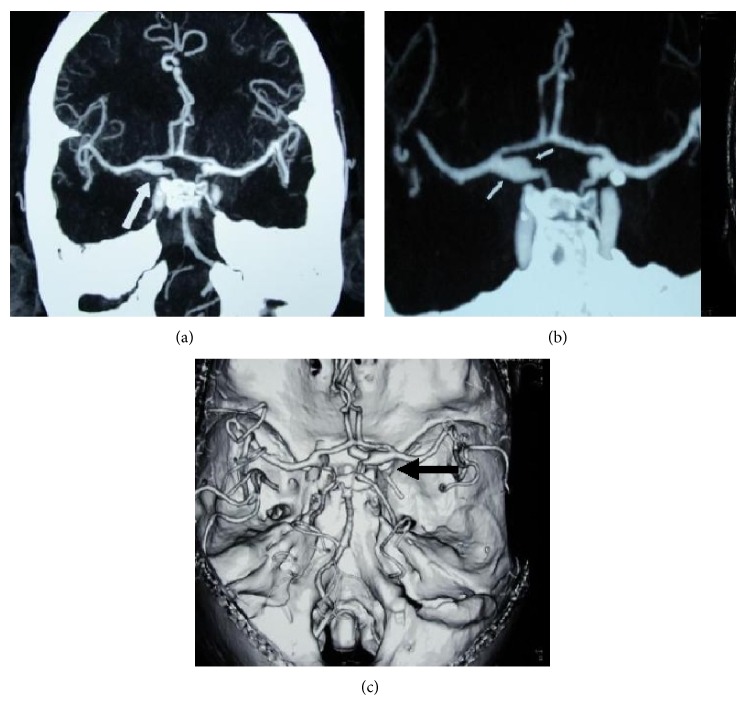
CTA brain showing PCom artery aneurysm. (a) CTA: an aneurysm arising from origin of right posterior communicating artery (PCom) (white arrow); (b) CTA: the aneurysm measured 11 mm in larger dimension with a broad-base/neck of 5 mm and a dome of 3.5 mm (white arrows); (c) vascular reconstruction: right PCom aneurysm on vascular reconstruction imaging (arrowhead) with prominent ipsilateral PCom and correspondingly small/hypoplastic ipsilateral P1 of PCA (posterior cerebral artery).
